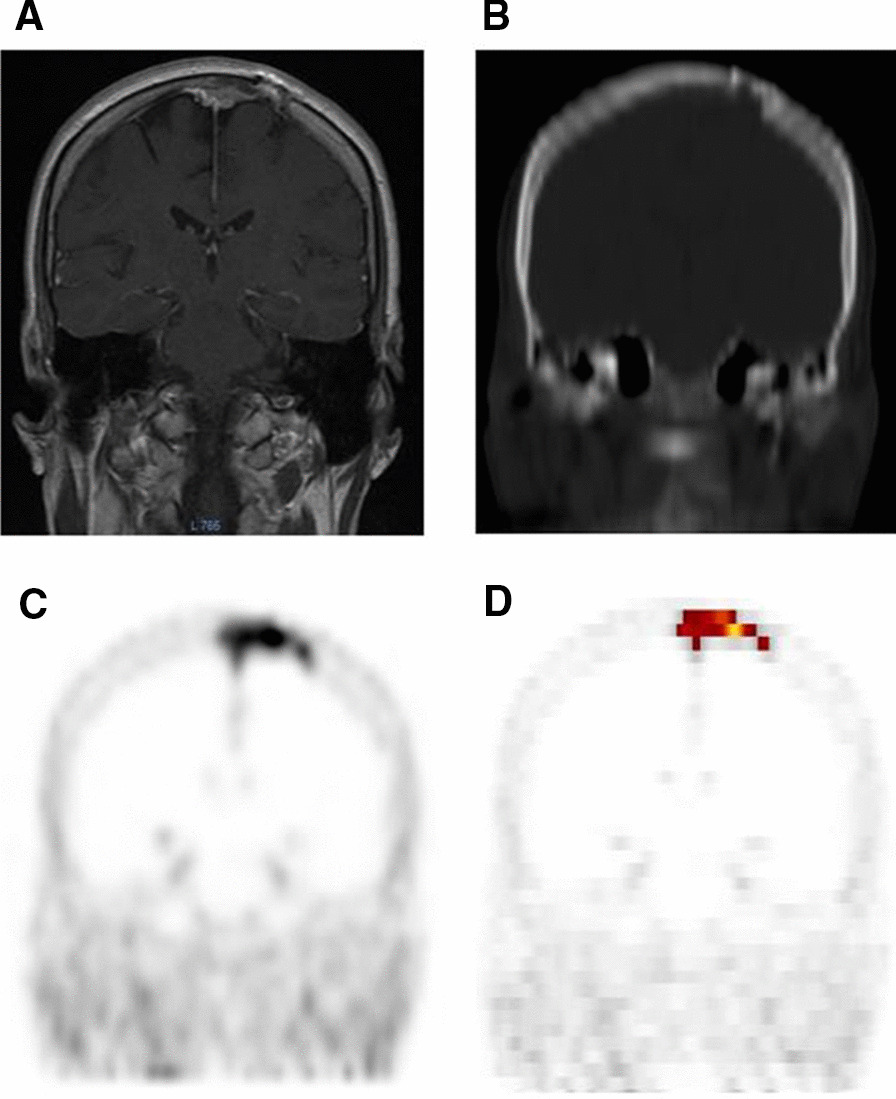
Left parietal meningioma with calvarial involvement. A Pre-treatment MRI, B Pre-treatment CT-scan, C. Pre-treatment 68 Ga-DOTATATE PET, D image from ROVER adaptive thresholding software
